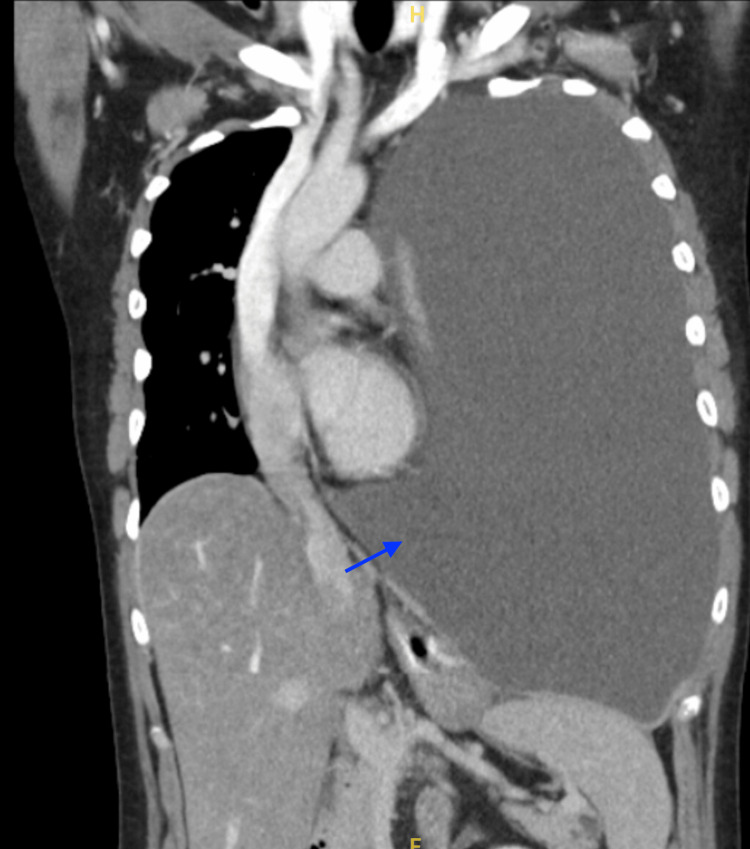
CT in coronal view, blue arrow pointing to a massive left pleural effusion occupying the entire hemithorax with total atelectasis of the left lung
